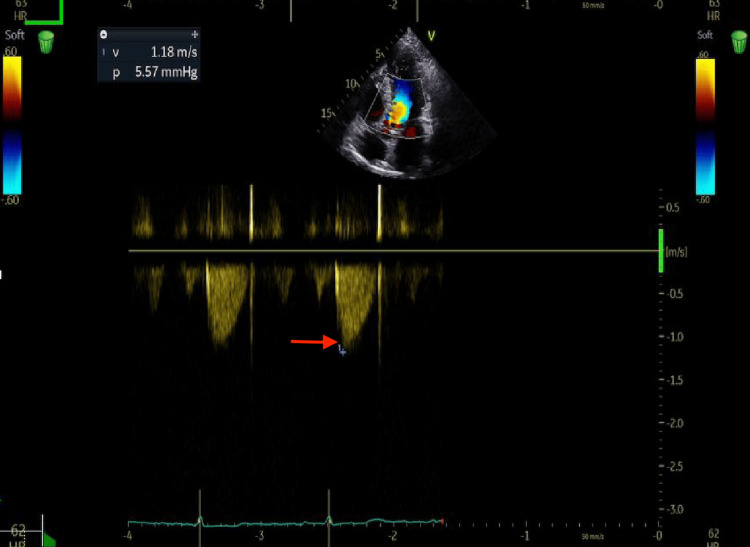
Follow-Up Continuous-Wave Doppler at Six Months Follow-up transthoracic echocardiography with continuous-wave Doppler demonstrating minimal residual LVOT dynamic obstruction at rest. The red arrow marks the peak of the systolic envelope, corresponding to a peak gradient of 5 mmHg at six-month follow-up. LVOT: Left ventricular outflow tract.
